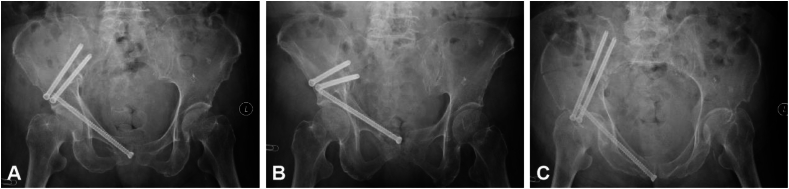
Postoperative radiographs to evaluate the reduction quality.
